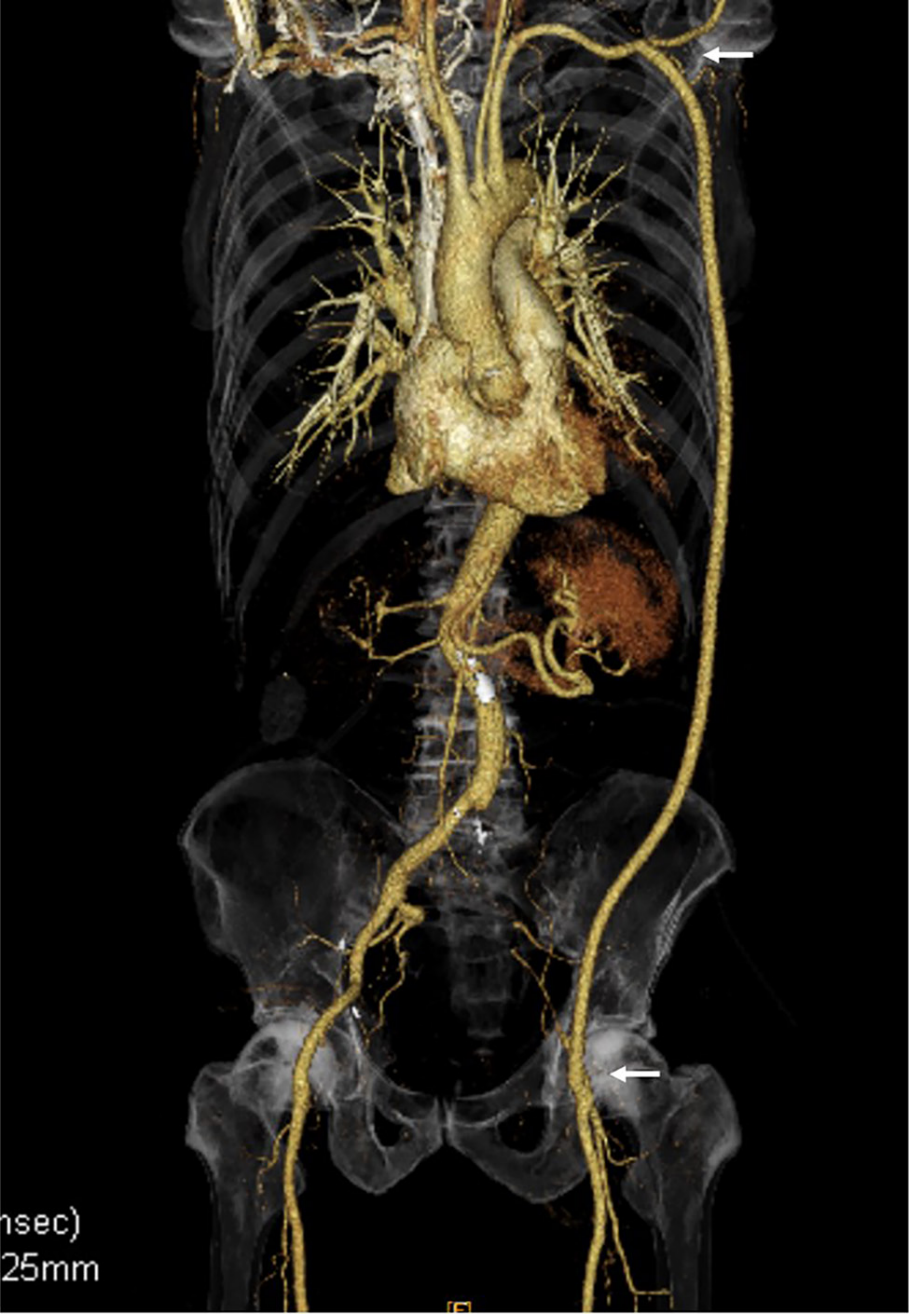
CT on postoperative day 7 revealed no issue at all, with patency of the axillo-femoral bypass.
